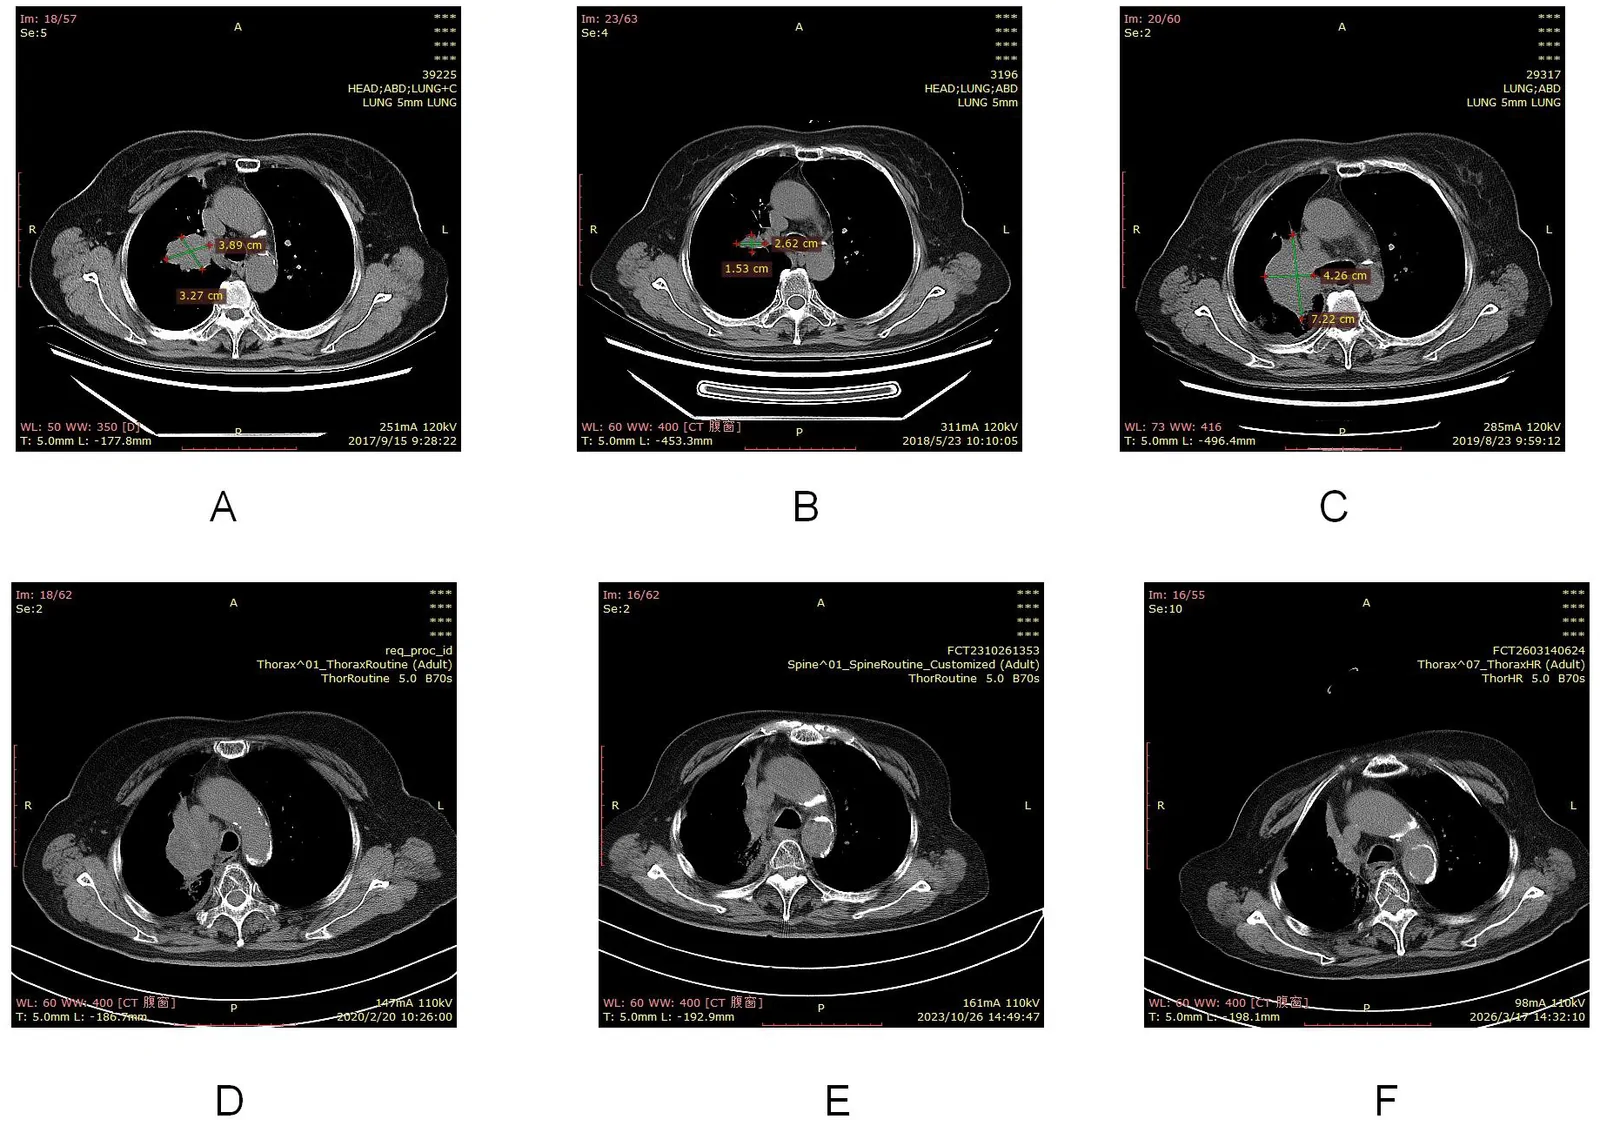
Serial chest computed tomography images during the disease course. (A) Baseline chest computed tomography in September 2017 showing a right hilar mass with mediastinal lymph node enlargement. (B) Post-treatment imaging after concurrent chemoradiotherapy showing PR. (C) Imaging at disease recurrence after subsequent chemotherapy. (D) Tumor regression after anlotinib treatment, before biopsy-confirmed extrathoracic progression. (E) Follow-up chest CT in October 2023 showing stable disease during anlotinib maintenance after discontinuation of sintilimab. (F) Latest available follow-up imaging in March 2026 showing ongoing SD.
